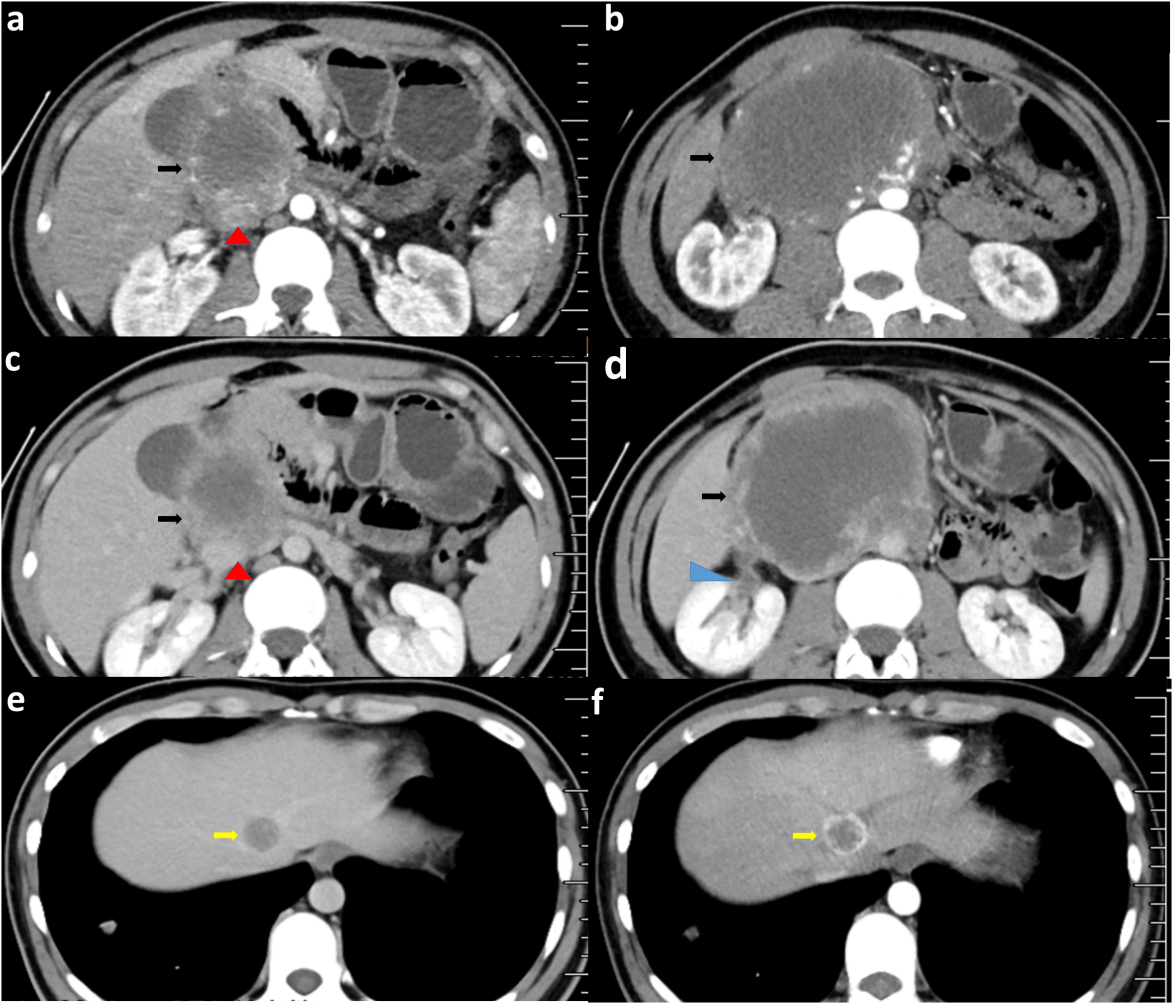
CTA of the abdominal aorta at patient admission. (A, B, F) show artery-phase images. (C, D, E) are venous-phase images. The black arrow points to the tumor; the red triangle points to the inferior vena cava (the boundary between the inferior vena cava and the tumor is unclear); the blue arrow suggests renal pelvis expansion changes. The yellow arrow points to the liver metastasis.
